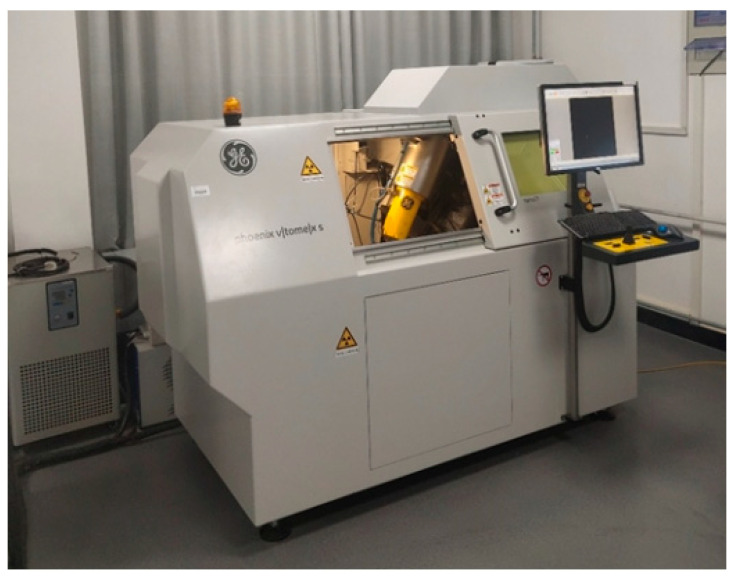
Computed Tomography CT System.
